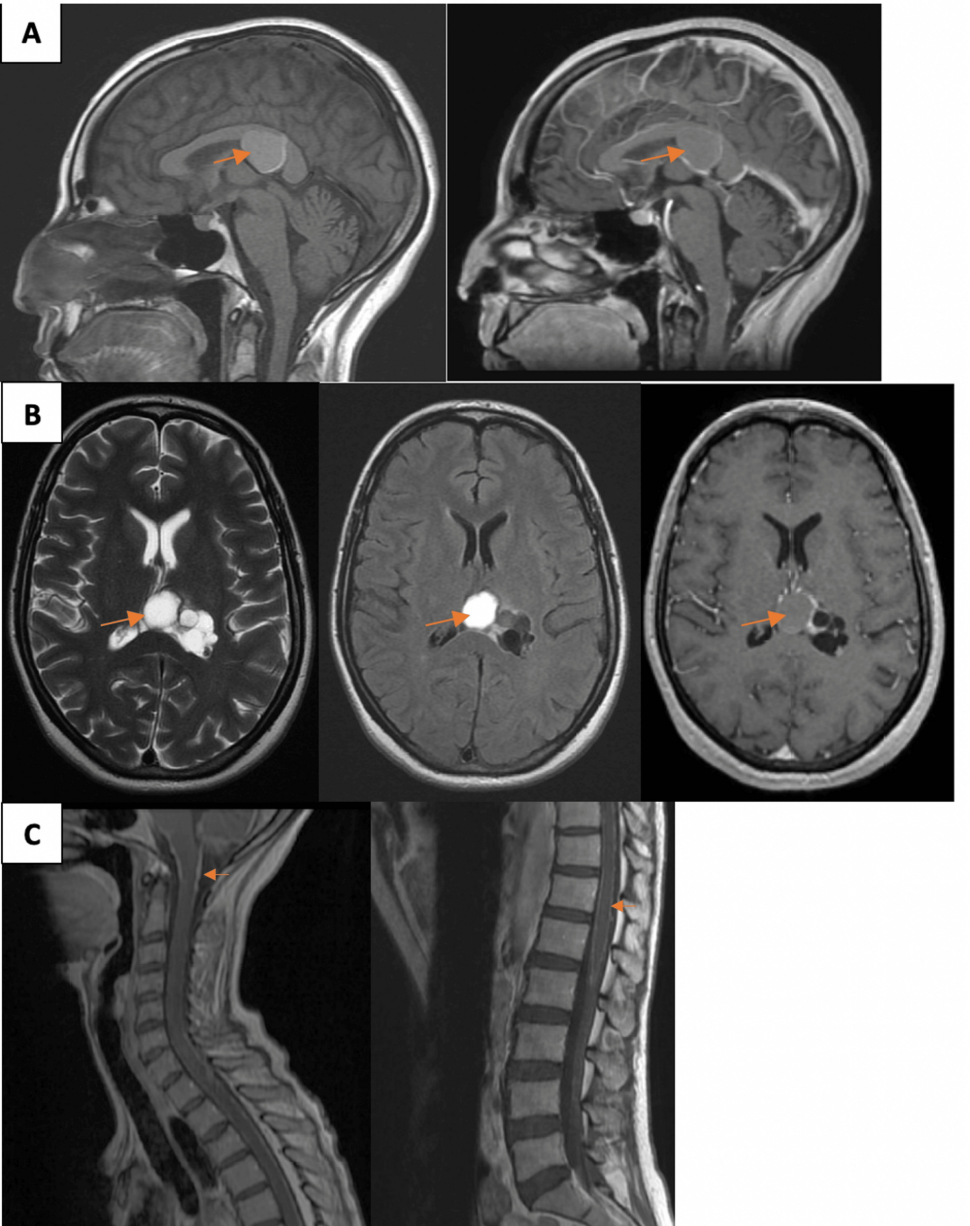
Pre-operative MRI scans of the patient. (A) Sagittal T1-weighted MRI brain without and with Gd-enhancement. (B) Axial T2-weighted, FLAIR, and T1-weighted MRI of the brain with Gd-enhancement exhibiting a complex multilobulated predominantly cystic lesion involving the velum interpositum and the trigone of the left lateral ventricle. (C) Sagittal T1-weighted MRI spine with Gd-enhancement exhibiting diffuse leptomeningeal enhancement. (MRI, magnetic resonance imaging; Gd, gadolinium).
